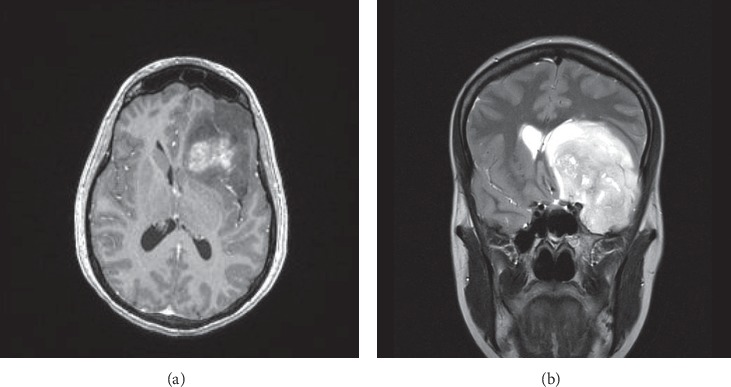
Brain magnetic resonance imaging, axial (a) and coronal (b) views, shows a large mass within the left anterior and middle cranial fossae with rightward midline shift on the underlying brain parenchyma (subfalcine herniation).
